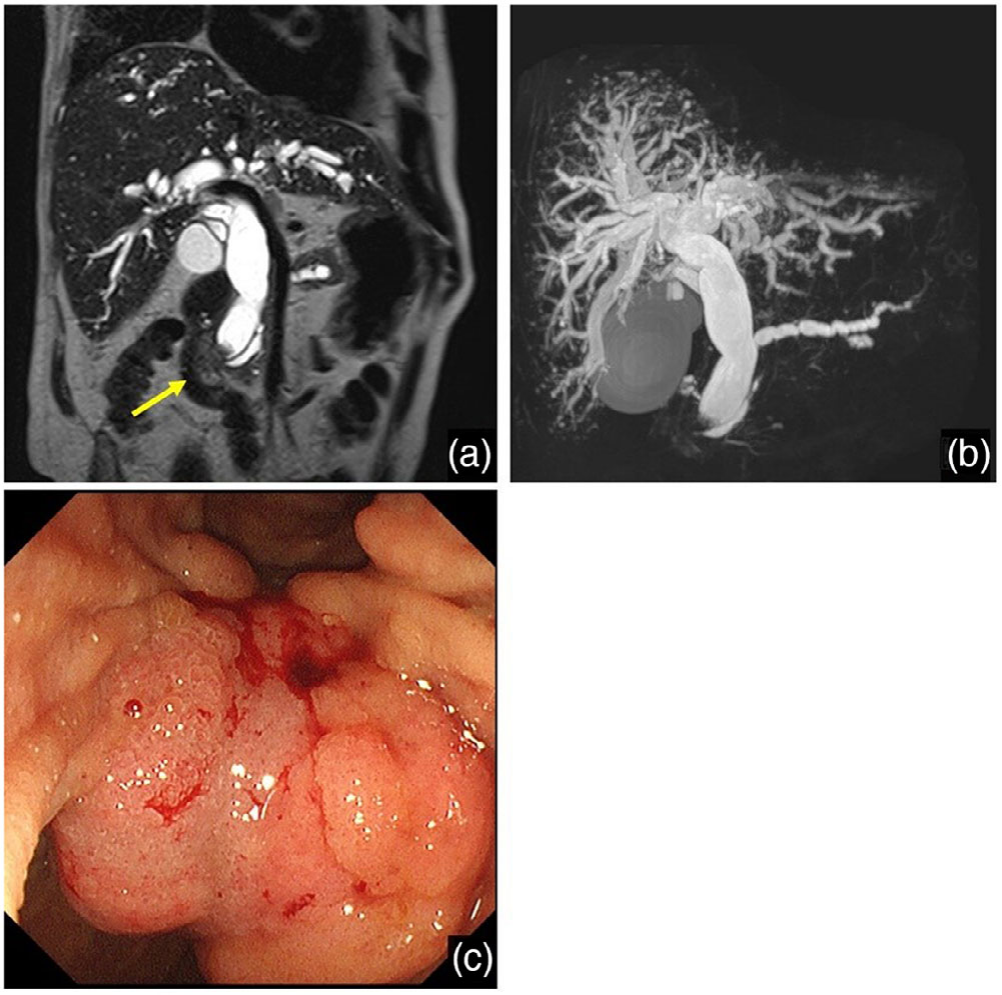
Imaging findings of ampullary carcinoma. (A) T2‐weighted magnetic resonance imaging (MRI) showing a tumor at the ampulla of Vater (yellow arrow) with biliary and pancreatic duct dilation. (B) Magnetic resonance cholangiopancreatography (MRCP) demonstrating dilation of the biliary tree due to ampullary obstruction. (C) Endoscopic view of the large ampullary tumor obscuring the biliary orifice, which prevented successful ERCP.
